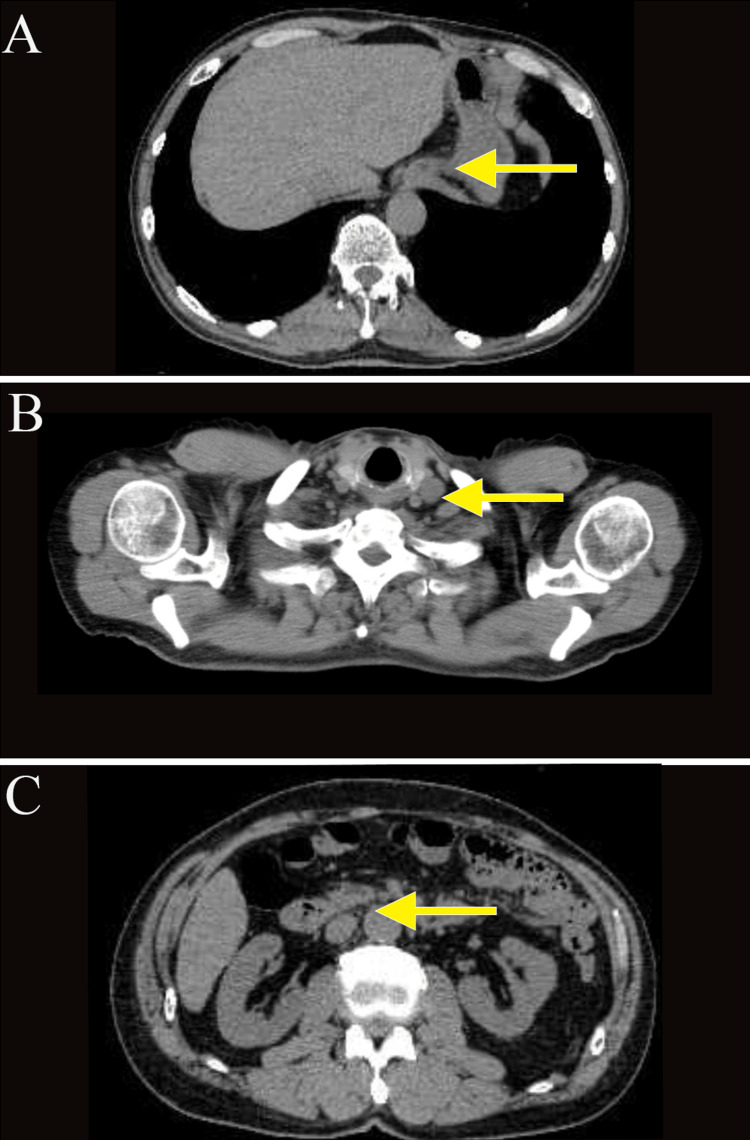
Computed tomography (A-C) Images taken after chemotherapy. CT scans showed that the tumor and multiple enlarged lymph nodes had almost disappeared.
